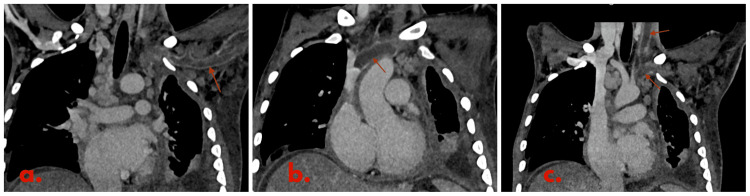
Coronal venous phase CT-image showing a hypodense non-enhancing filling defect causing non-opacification of the left visualized distal axillary vein (a), left subclavian vein (b), left internal jugular vein and left brachiocephalic vein and extension into the SVC (c). SVC: superior vena cava.
